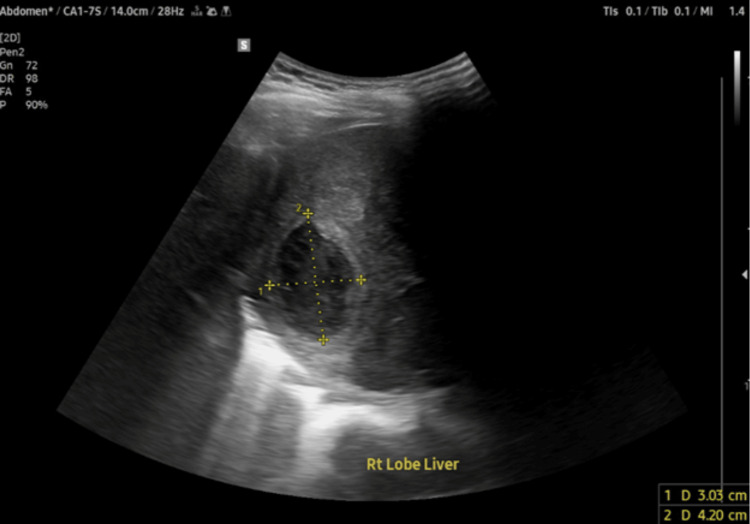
Follow-up abdominal ultrasound showing the right hepatic lobe drainage stent in situ and an interval regression in the size of the hepatic abscess, with measuring calipers indicating the residual lesion is 3.03 cm x 4.20 cm
